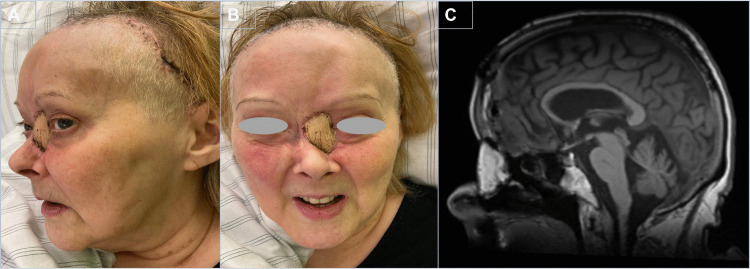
Postoperative outcome. (A) Well-healed reconstruction with restored contour. (B) Fully viable flap without vascular compromise. (C) MRI demonstrating typical postoperative appearance
